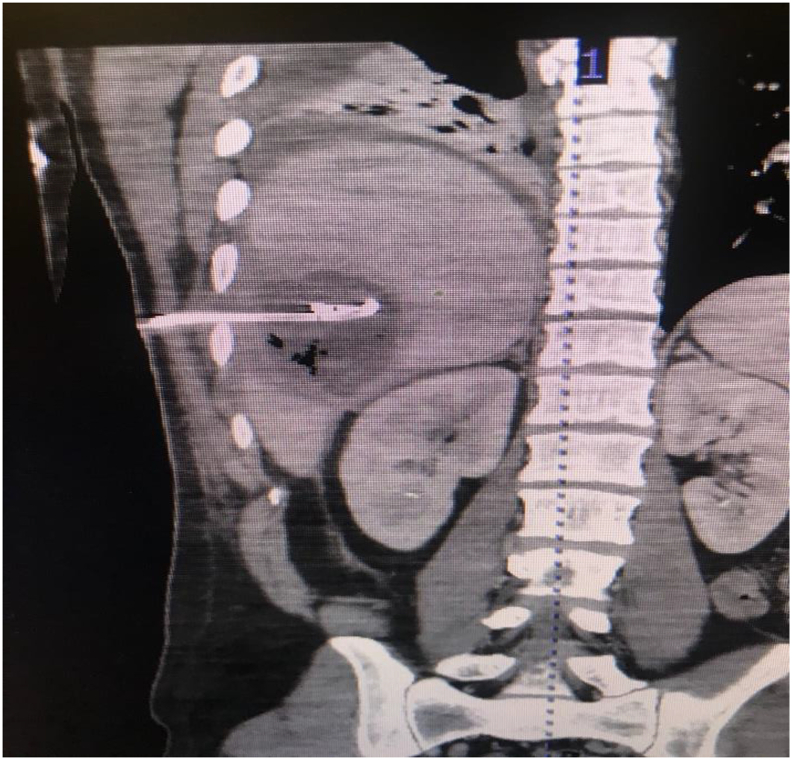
Coronal CT showing hepatic abscess with drains.
